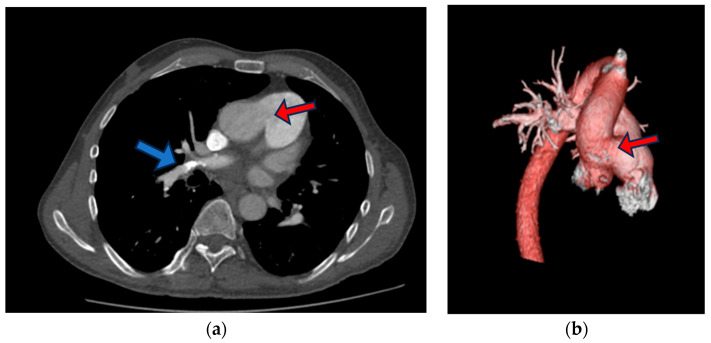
Chest CT (a) slide that shows aortopulmonic window (red arrow), right upper lobe anterior segment sub-segmental branches with small filling defects with adjacent calcification (blue arrow), (b) three-dimensional (3D) reconstruction of contrast-enhanced CT angiography images of the thoracic aorta and pulmonary artery trunk showing aortopulmonic window (red arrow).
